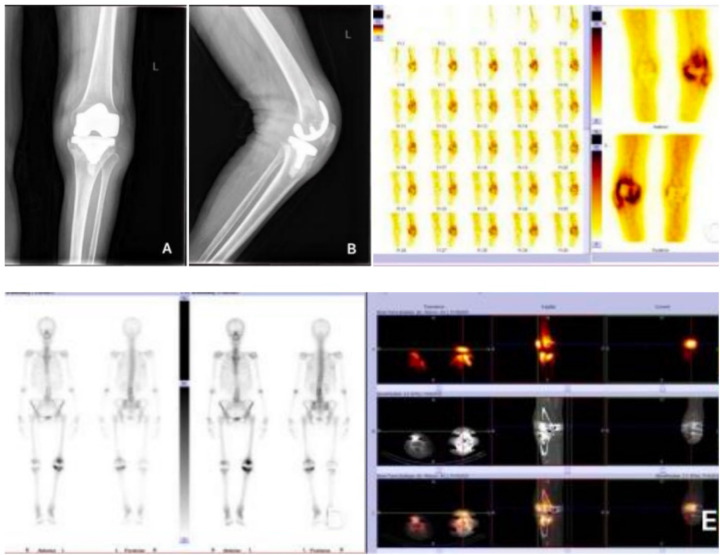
(A,B) Anteroposterior and lateral x-ray films of the left knee joint 10 years after total knee arthroplasty; (C) dynamic imaging of both lower extremities; (D) whole-body bone scan; (E) SPECT/CT tomographic fusion imaging.
